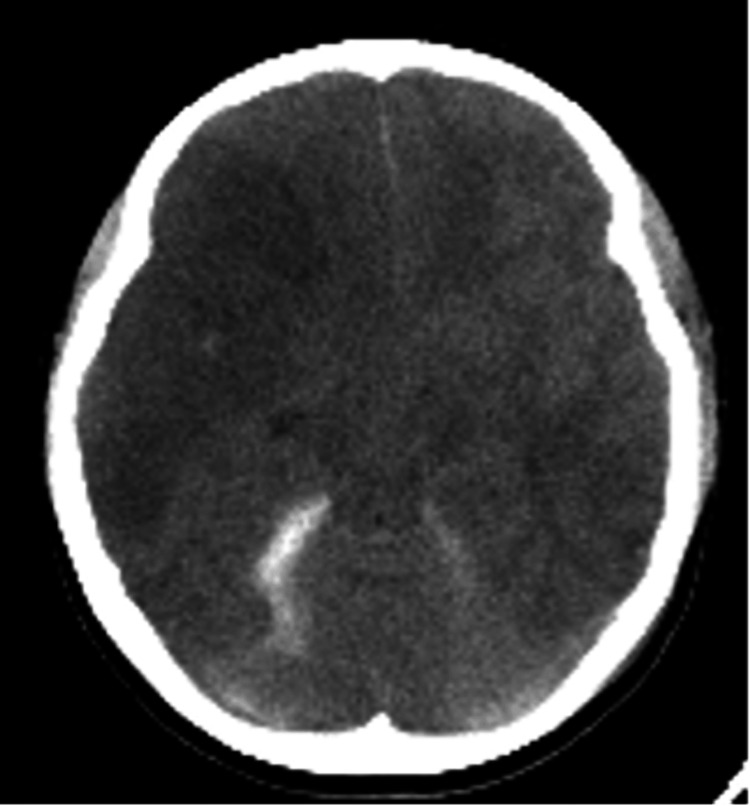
A CT scan of the head on the seventh day from the onset showed extensive ischemic lesions in the right middle cerebral artery territory and left rectal gyrus. CT: computed tomography
